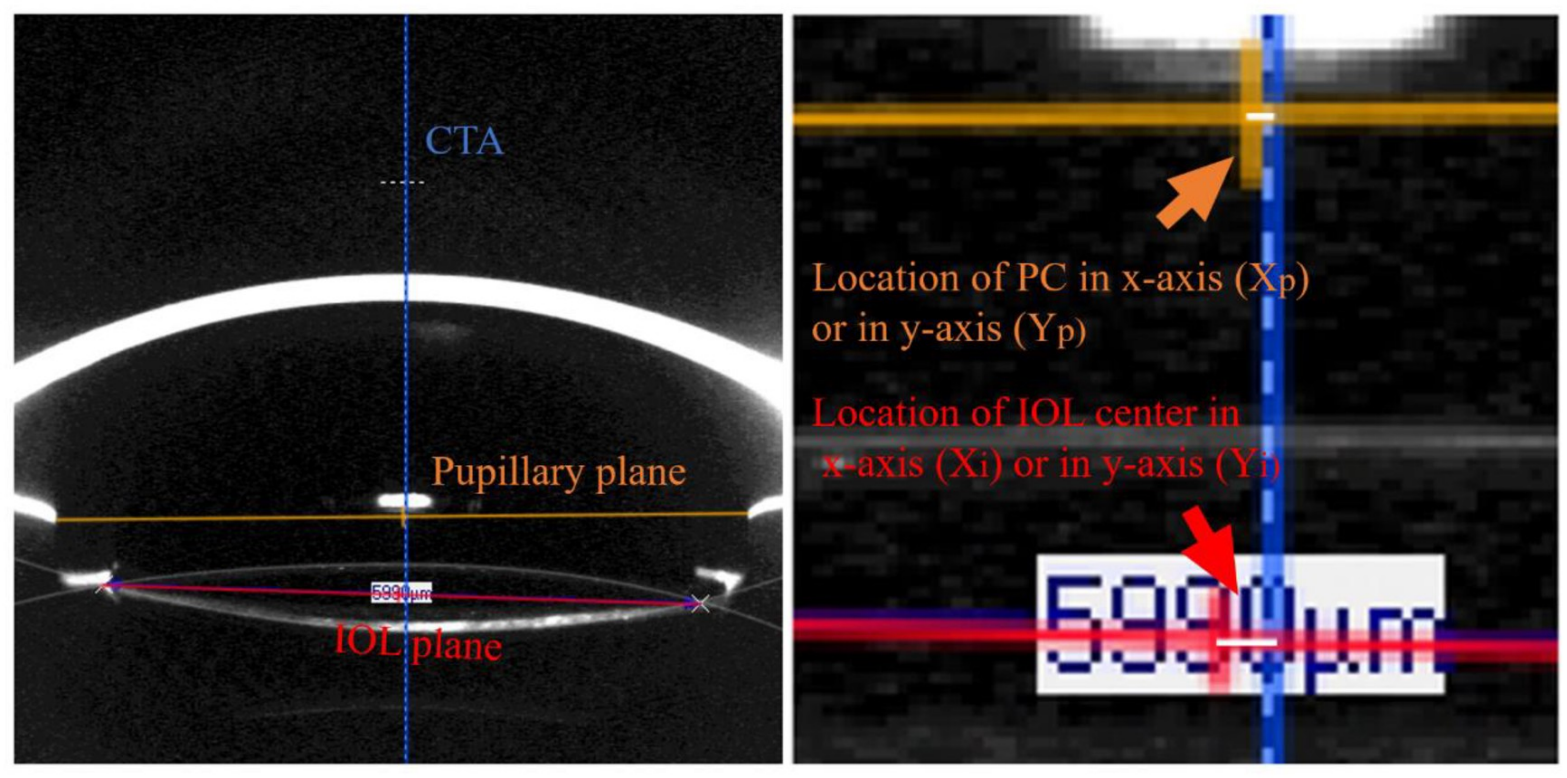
Definition for all parameters in Sheimpflug image using Pentacam. CTA, corneal topographic axis; IOL, intraocular lens; PC, pupil center. The blue line represents CTA. The orange line represents pupillary plane. The red line represents IOL plane.
